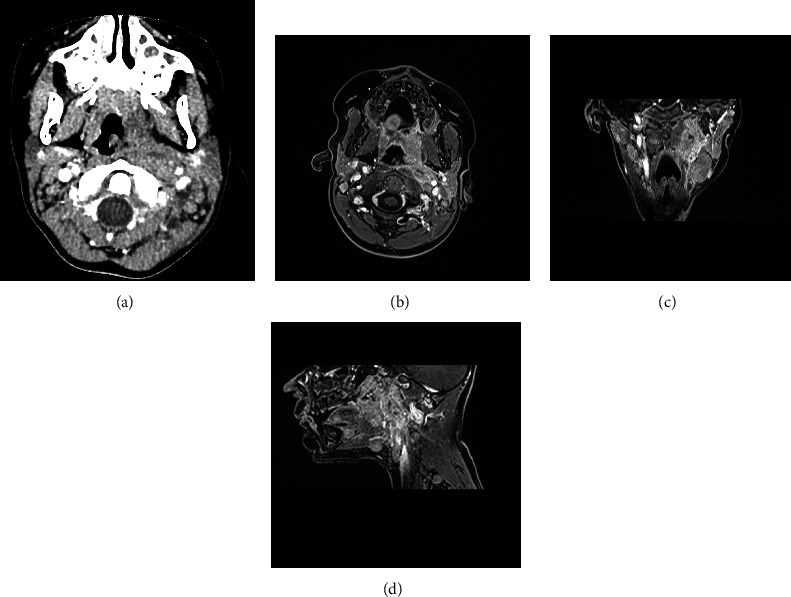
(a) Head and neck CT scan showing retropharyngeal soft tissue swelling and central low-density area, without rim enhancement. (b–d) Head and neck MRI (contrast enhanced T1) showing tissue enhancement with a central hypodense area of left deep parapharyngeal space near carotid space.
